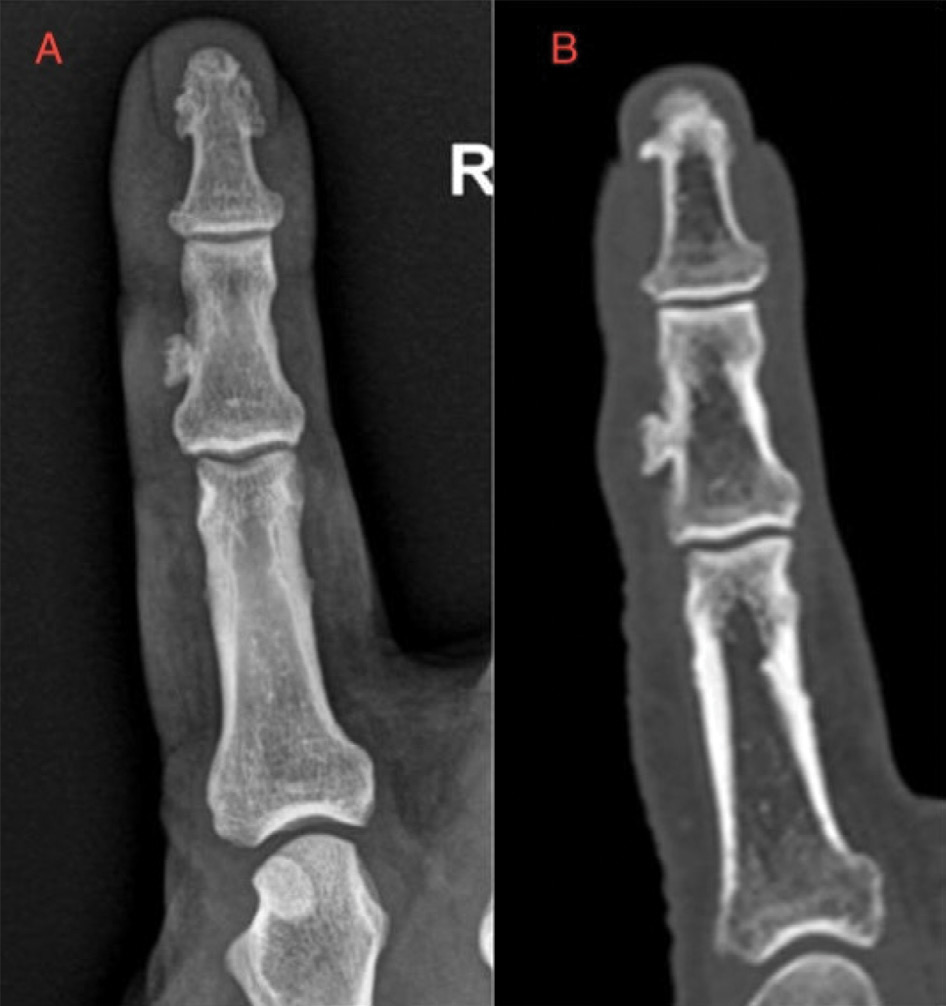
Lesion on radiography (A) and on computed tomography (B).
